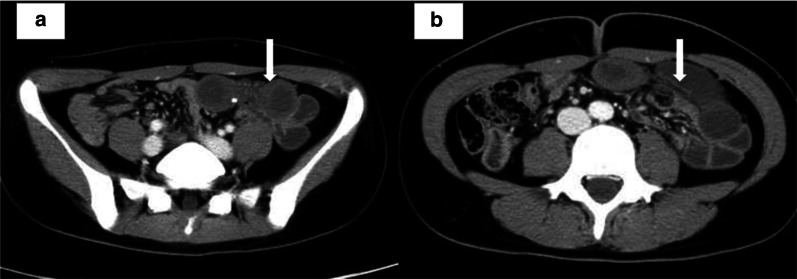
Contrast-enhanced CT findings. Contrast-enhanced CT revealed submucosal edema of the intestinal wall (arrow) and a sustained contrast effect (a). A whirl sign (arrow) was present, and strangulated small bowel obstruction was suspected (b)
